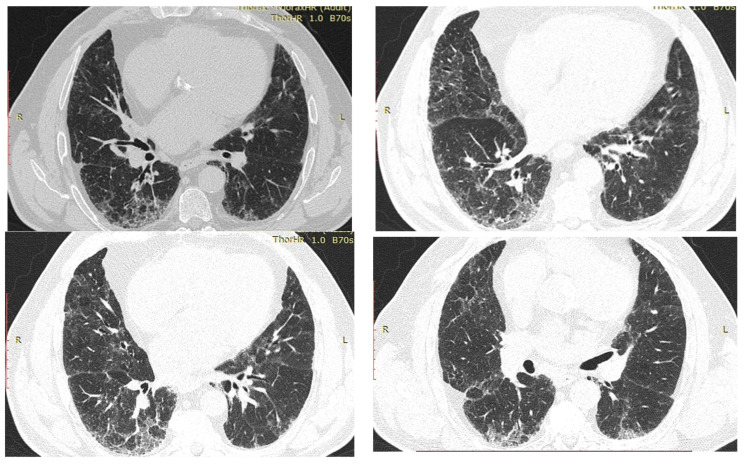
HRCT—2021—Case 2. Reticulation plaques, traction bronchiectasis, subpleural air microcysts. No honeycombing. Probable UIP pattern.
